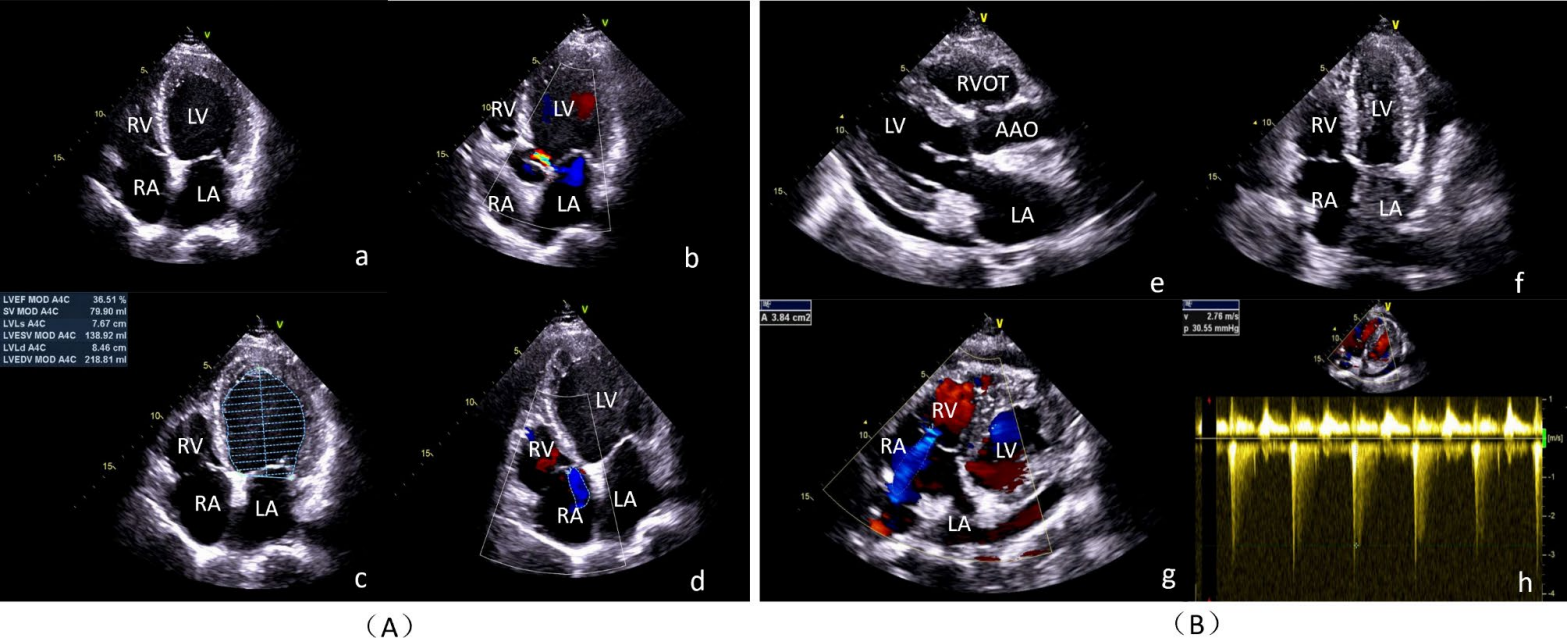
Ultrasound Doppler before (A) and after(B) heart transplantation. LV: left ventricle; LA: left atrium; RV: right ventricle; RA: right atrium; RVOT: right ventricular outflow tract; AAO: ascending aorta; LVEF: left ventricular ejection fraction
